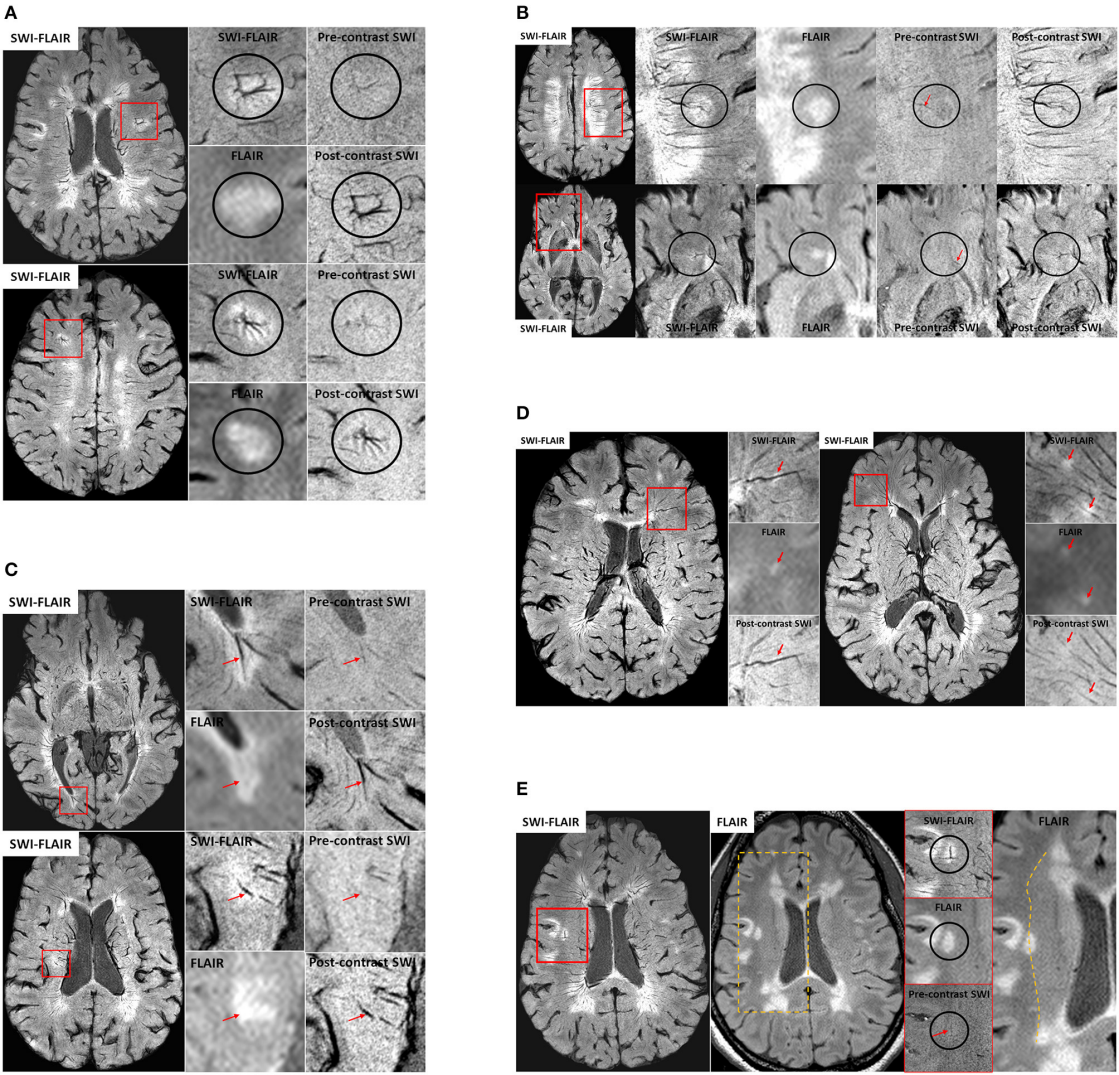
(A) Examples of venous angioma like abnormalities in MS lesions from two patients. The small vessels joining the lesion-centric junction are not visible in conventional imaging and hence these DVAs have gone unrecognized in the past. Only the primary draining veins were slightly visible on the pre-contrast SWI data, confirming their venous origin. (B) Two different examples of micro-angiomas in MS lesions. Unlike the cases for larger angiomas, the pre-contrast SWI does not show any hints of these smaller vessels seen on the post-contrast SWI or SWI-FLAIR data, except for the primary vein (red arrows) that is draining the lesion-centric spoke-like small vessels. (C) Two different veins exhibiting dilation with the MS plaques. SWI-FLAIR data clearly shows the abrupt change in the vein diameter within the lesion. If these are active lesions, the inflammation may continue to develop around these vessels over time. In these cases, there may not be any further gadolinium enhancement as the lesion grows due to reduction of the vessel size or the complete blockage due to collagenosis. (D) Small ovoid WMHs (red arrows) located along the vessel wall of the small vessels as confirmed on SWI-FLAIR, FLAIR and post-contrast SWI data. (E) An example case showing a potential anastomotic vein. Once again, the pre-contrast SWI data was used to confirm the venous origin (red arrow) of this lesion-centric anomaly. The medial-to-lateral change in WM intensity on the FLAIR data (dotted line on the right-most image) is suggestive of the presence of the corona radiata layer in the WM and note that the anastomotic vein is abutting at this junction.
